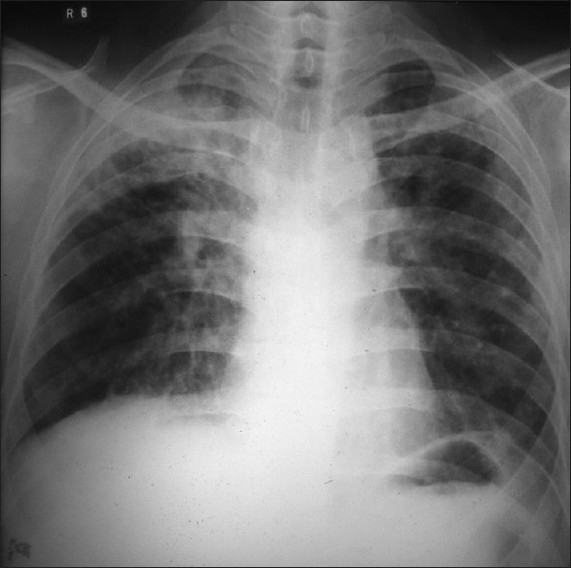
Chest X-ray (1998) shows right tubercular exudative lesions with cavity in right upper lobe
